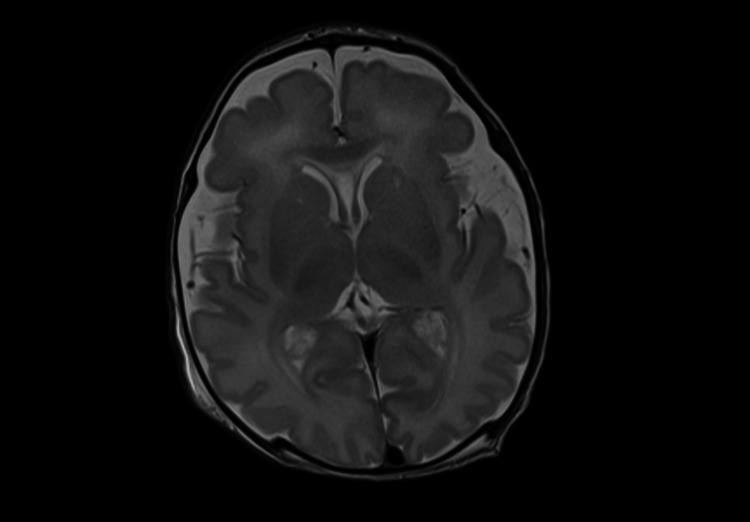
Axial T2-Weighted MRI of the Brain Performed on Day 5 of Life, Demonstrating Normal Brain Morphology With No Evidence of Structural Abnormalities, Hemorrhage, or Diffusion Restriction.
